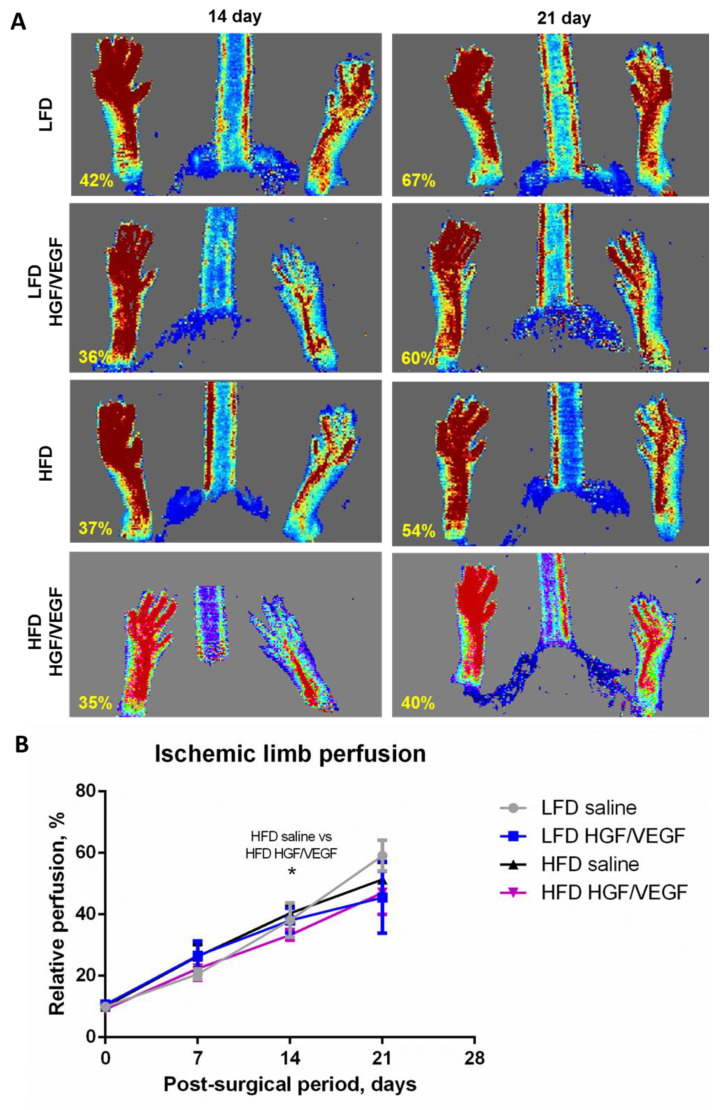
HGF/VEGF* plasmid injection induces a transient delay of blood flow recovery in HFD group. (10 + 3 weeks of experiment). (A) representative Laser Doppler scanning images subcutaneous blood flow in experimental groups; (B) dynamics of blood flow recovery after *HGF/VEGF* therapy under LFD or HFD dietary intervention. Data is presented as mean ± SEM; Kruskal–Wallis test with post hoc Dunn’s test; * *p* < 0.05.
